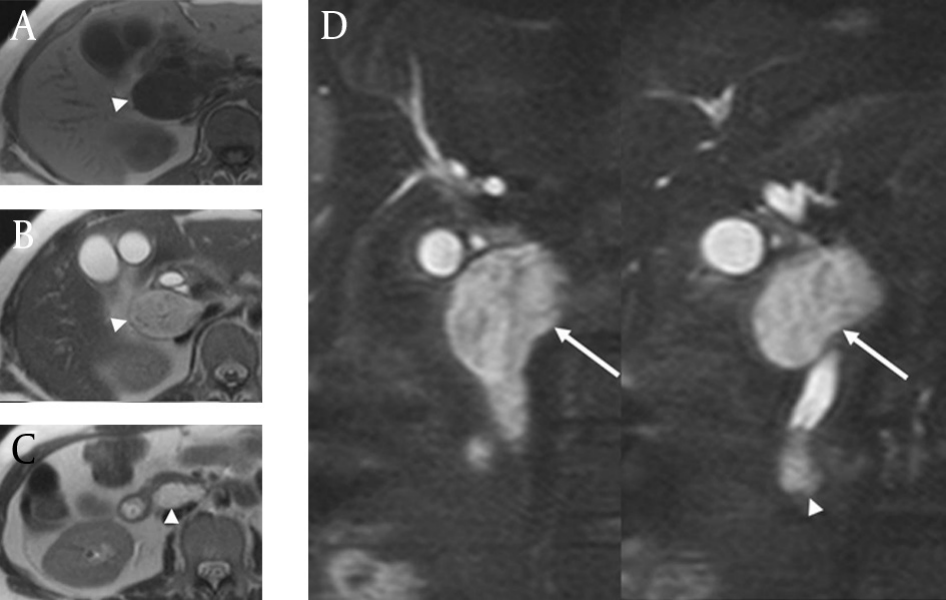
Figure 3. T2-weighted (half-Fourier acquisition single-shot turbo spin-echo, HASTE) images on 3T MRI. A, Axial T1-weighted image shows a homogeneous hypo-intense mass (arrowhead) in the portocaval area. B, C, Axial images show well-circumscribed heterogeneous hyper-intense masses (arrowheads) in the portocaval area and behind the uncinate process of the pancreas. D, Coronal images show the elongated mass seen in A (arrows) extending from behind the primary EHD lesion with internal hypo-intense septa positioned along the hepatoduodenal ligament. The primary lesion of the distal EHD also exhibited higher internal signal intensity (arrowhead) than typically seen in adenocarcinoma.
